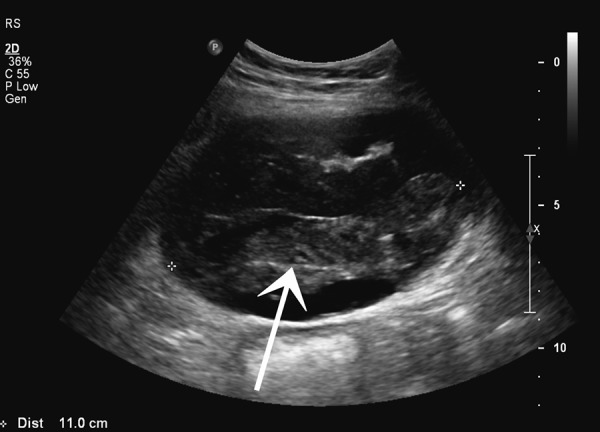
Ultrasound showing subcapsular hematoma causing direct compression of renal parenchyma (arrow).
